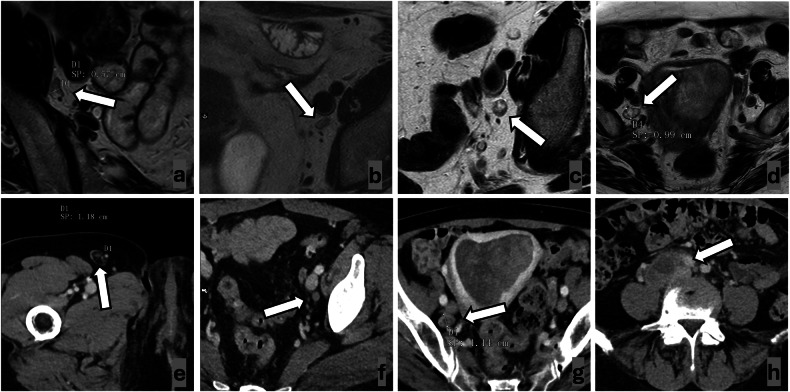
Examples of lymph nodes’ (arrows) morphological feature evaluation on high-resolution T2-weighted MR images (a–d) and on portal venous phase CT reconstructions (e–h). An ovoid shape can be observed (a, b, d, e, g, h), whereas a round shape is shown in (c, f). A preserved fatty hilum is present (a, e). Irregular margins (b, f, g, h). Heterogeneous signal intensity (b, c, d, g, h), with focal necrosis (c, g), and gross necrosis (d, h) (notably, d, g show the same lymph nodes, but the amount of necrosis is better appreciated on MRI than on CT)
